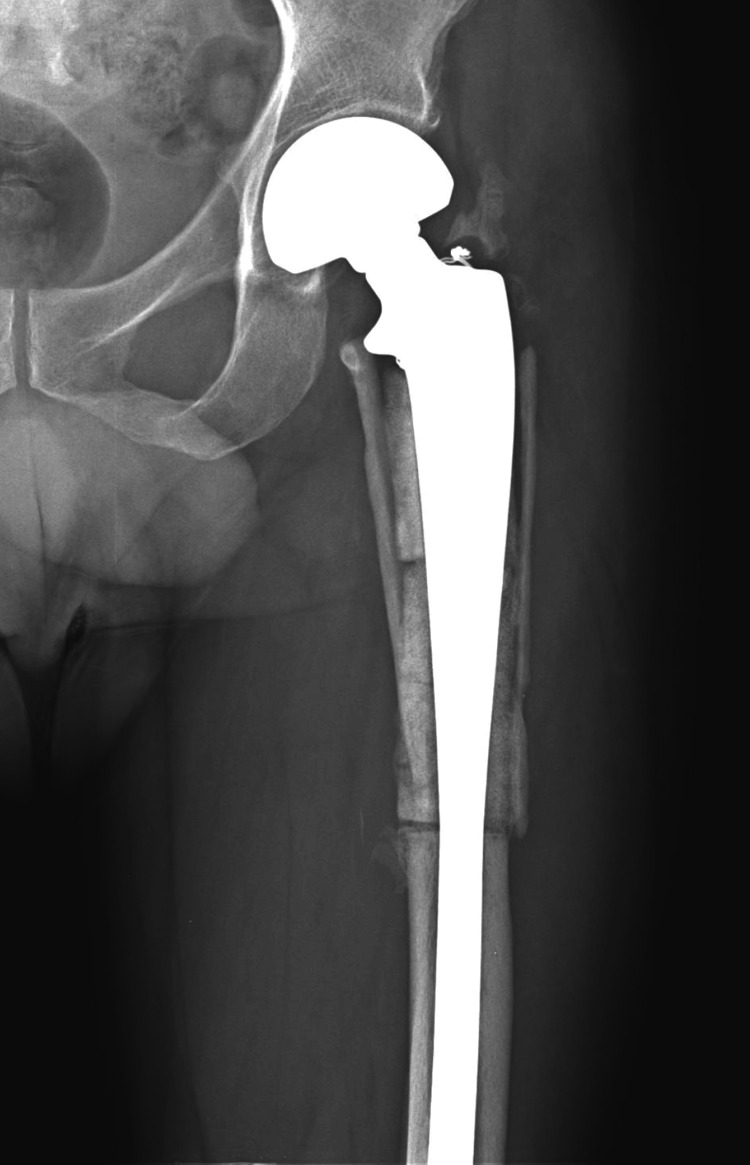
The radiodensity of the ceramic in a radiograph at one year
